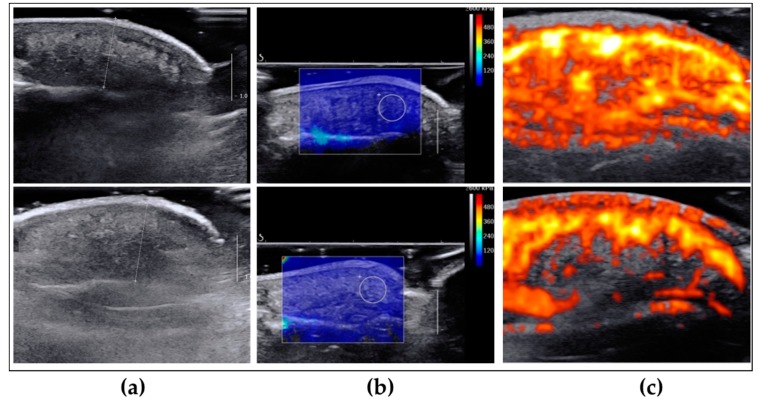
Representative echo-doppler images of regenerated (upper row) and un-injured collateral (lower row) fingertips. (a) Ultrasound b-mode imaging for the measurement of the pulp thickness; (b) b-mode imaging with superimposed share wave mapping for the measurement of the pulp elasticity. The white circle has a diameter of 5 mm; (c) b-mode imaging with superimposed power Doppler for the measurement of vascularity.
